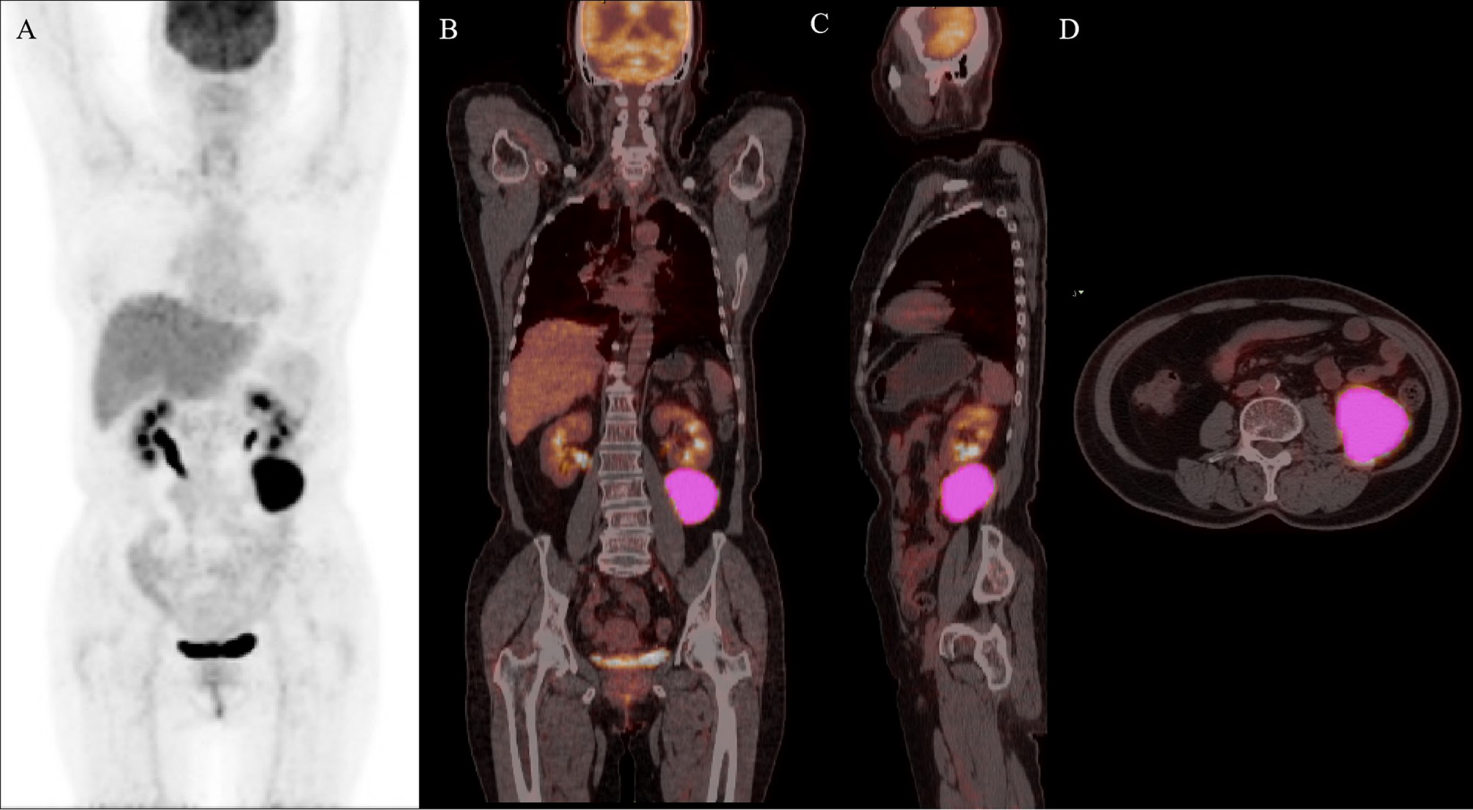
Maximal intensity projection image (A), coronary image (B), sagittal image (C), and transaxial images (D) of ^18^F-FDG PET/CT showing an example of VOI for measuring imaging features of BCL. A 68-year-old woman with stage IV DLBCL. MIP image showing metabolically active left abdominal lesion. 3D VOI was manually drawn with the LIFEx segmentation tool using the previously recommended SUV_max_ threshold of 41%.
